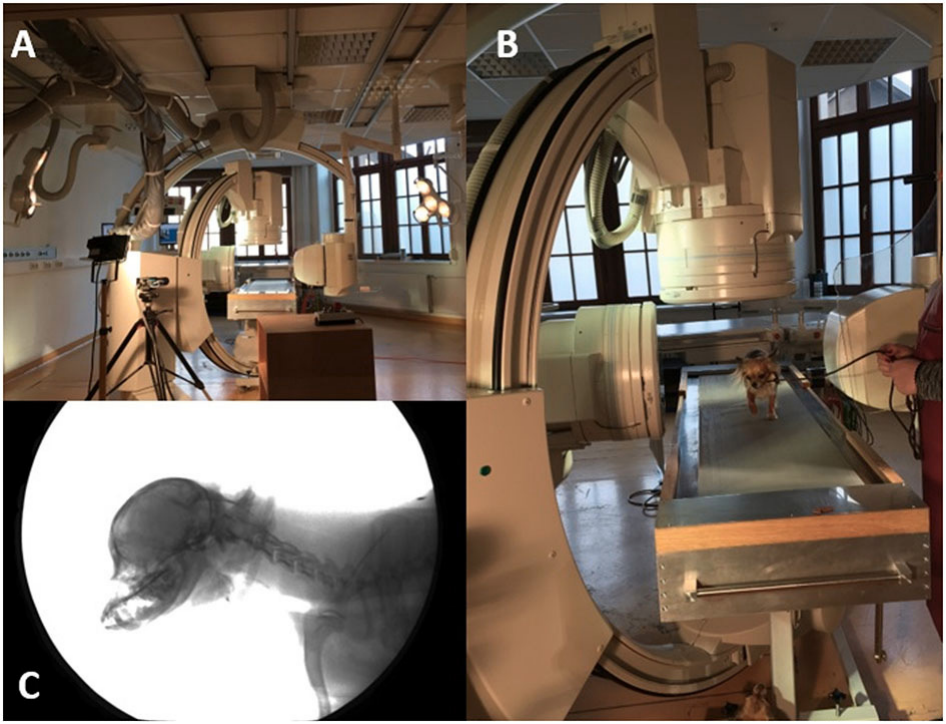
Fluoroscopy 90° experimental setup. (A): 90° fluoroscopy setting to record the-laterolateral and ventrodorsal beam path. The treadmill is located in the center. (B): Chihuahua walking on the treadmill located in the beam path. (C): X-ray video view of the laterolateral beam path.
